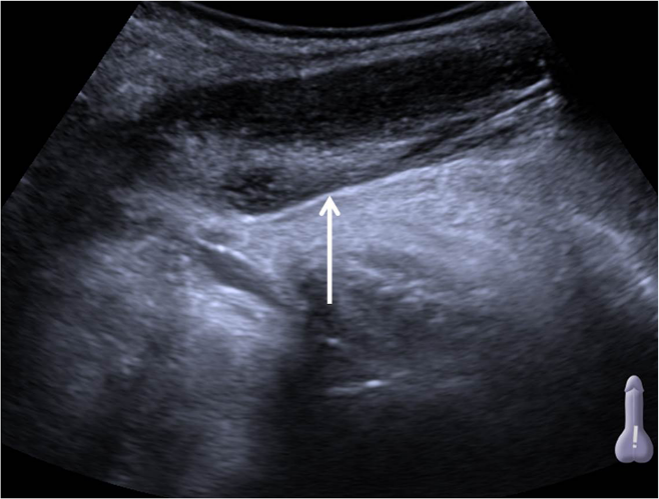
Ultrasound scan (longitudinal axis) of the right corpus cavernosum. Arrow shows thrombus in the proximal part.
